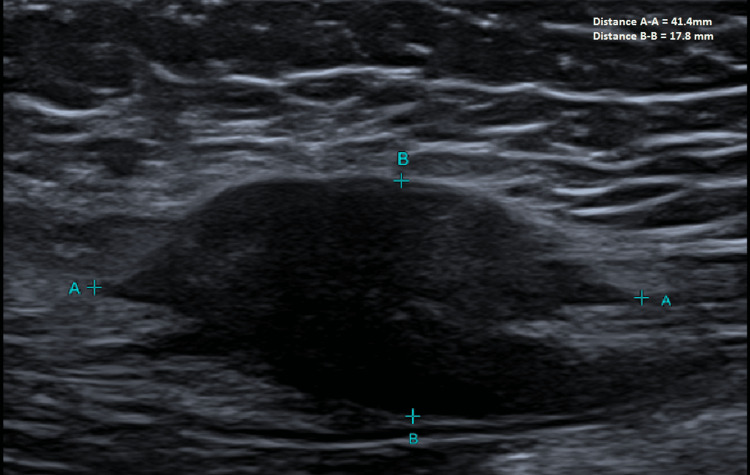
Ultrasound scan of the left abdominal wall demonstrating a hypoechoic mass within the soft tissue of the abdominal wall.
